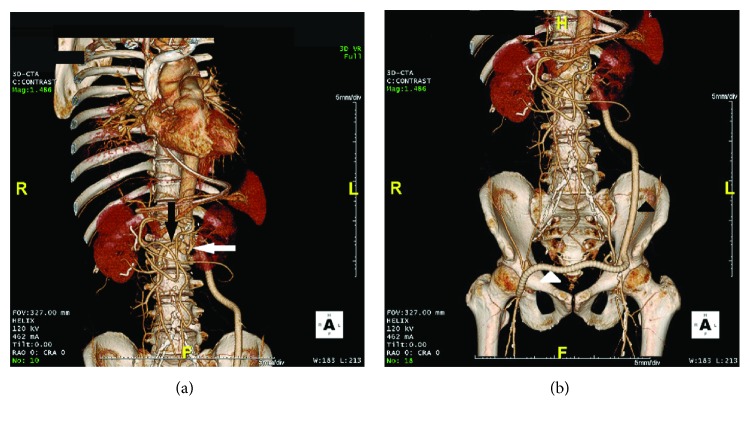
Postoperative three-dimensional reconstructed computed tomography scan shows the graft of the thoracoabdominal aorta and the additional femorofemoral bypass graft. (a) The superior mesenteric artery and right renal artery (black arrow) and the left renal artery (white arrow) were reconstructed with three of four leg grafts. (b) The left common femoral artery was bypassed with the remaining left leg graft (black arrowhead). The right common femoral artery was also bypassed with an 8 mm graft (white arrowhead).
